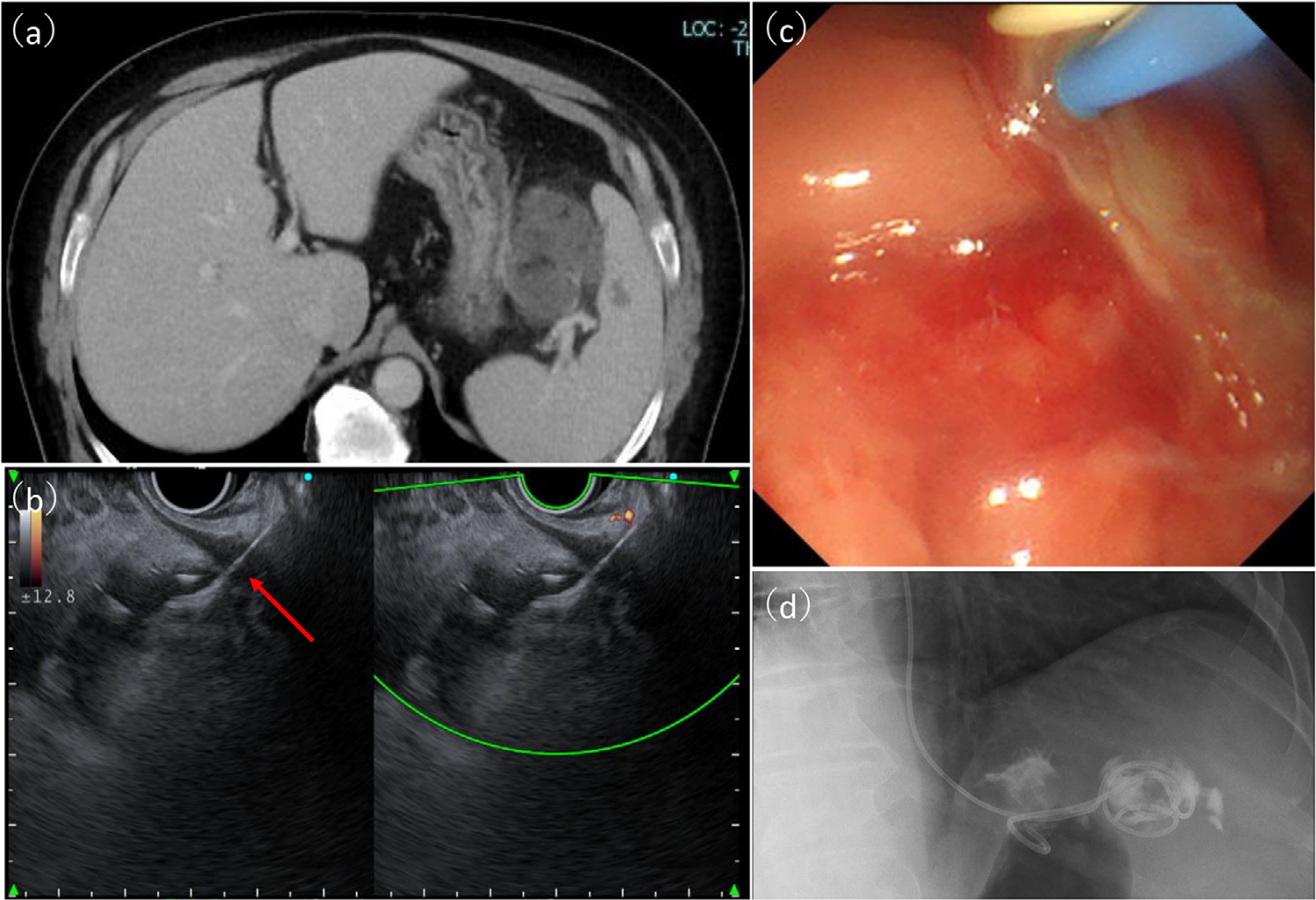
(a) Computed tomography image showed the increasing size of the abscess. (b) Endoscopic ultrasound image showing the puncture (red arrow) of the fluid collection using a 19‐G needle. (c) Drainage procedure showing the outflow of turbid, viscous, purulent material from the abscess cavity. (d) Placement of a 6Fr nasobiliary drainage tube and a 7Fr double‐pigtail stent in the abscess cavity.
